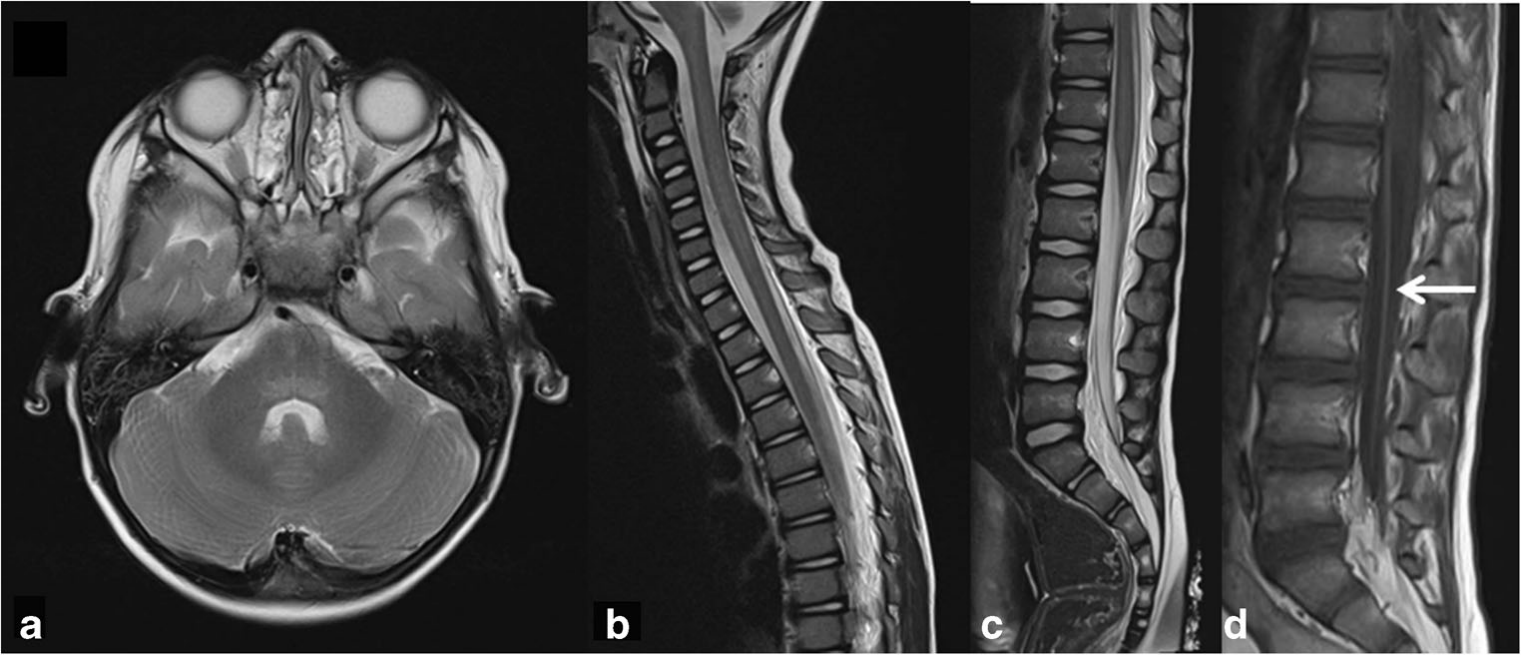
MRI of the neuraxis in a 3-year-old boy with EV-D68–associated AFM. a Brain: transverse T2-weighted image showing an area of slight hyperintensity in the dorsal pons (arrow). b and c Spinal cord: sagittal T2-weighted images showing longitudinal slight hyperintensity largely restricted to the central cord, where the gray matter is situated (arrow). d Spinal cord: contrast enhancement of the ventral caudal roots on a sagittal T1-weigthed image (arrow) (republished with permission from [16])
